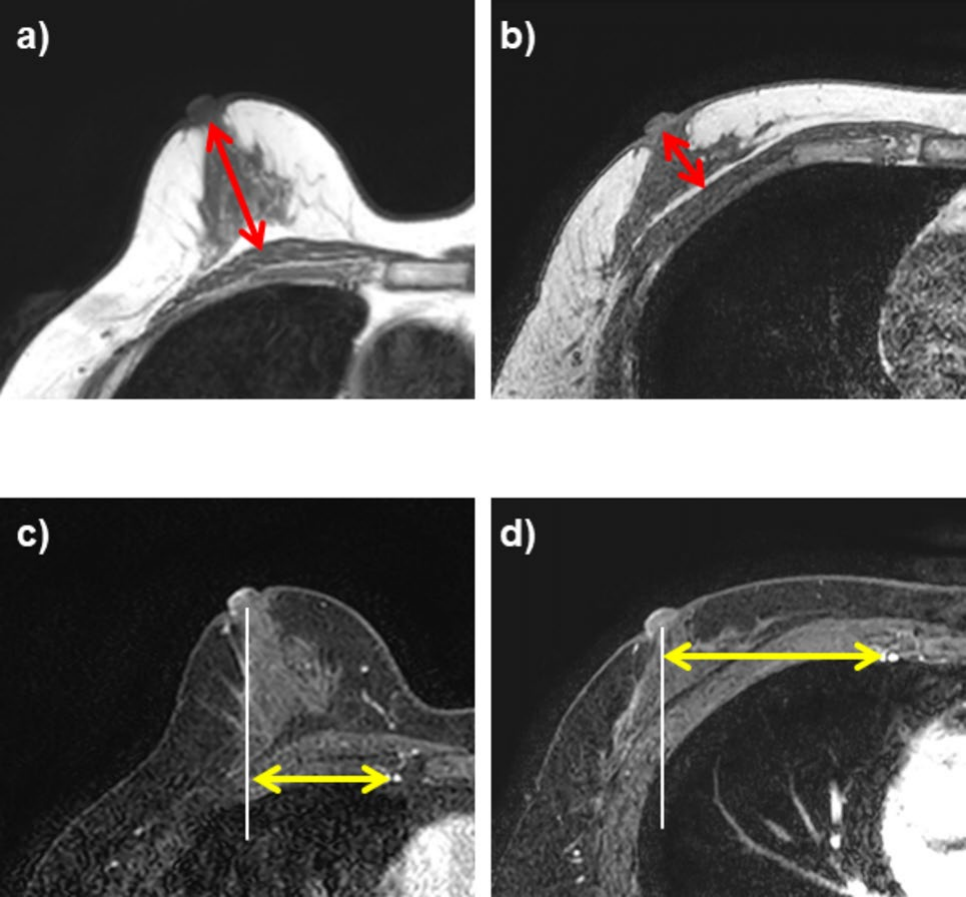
The minimal distance from the nipple to the pectoralis major both in the prone (a) and supine MRI (b). The degree of breast shape changes in the anterior–posterior direction is defined as the difference in the minimal distances between the two positions. The distance from the nipple to the internal thoracic artery in the prone (c) and supine breast MRI (d). The distance is measured as that between the vertical line from the center of the nipple base (white line) and the internal thoracic artery (yellow arrow). The degree of breast shape changes in the lateral direction is defined as the difference in the distance from the nipple to the internal thoracic artery between the two positions
